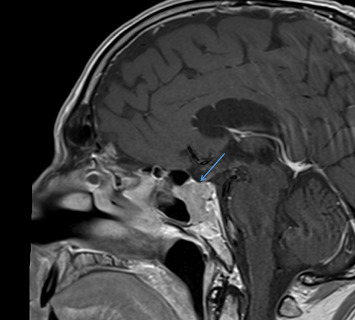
Sagittal MRI brain with and without contrast. Blue arrowhead indicates anterior sella mass involving the anterior sella and clivus without optic chiasm compression with extension to the level of the cavernous sinuses (picture taken with consent).
